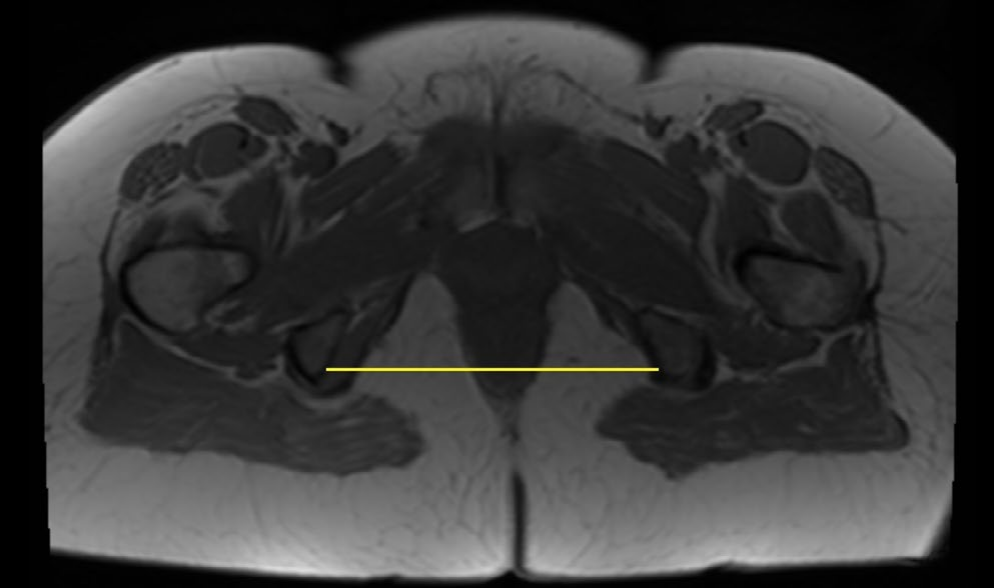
Magnetic resonance imaging (MRI) image with marked intertuberous distance (yellow line).
